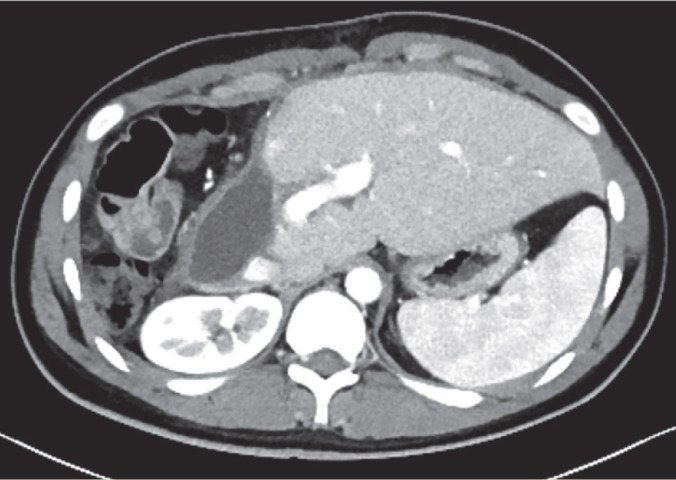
The axial CT image shows a collection consistent with a bilioma on the cross-sectional surface of the remnant liver
